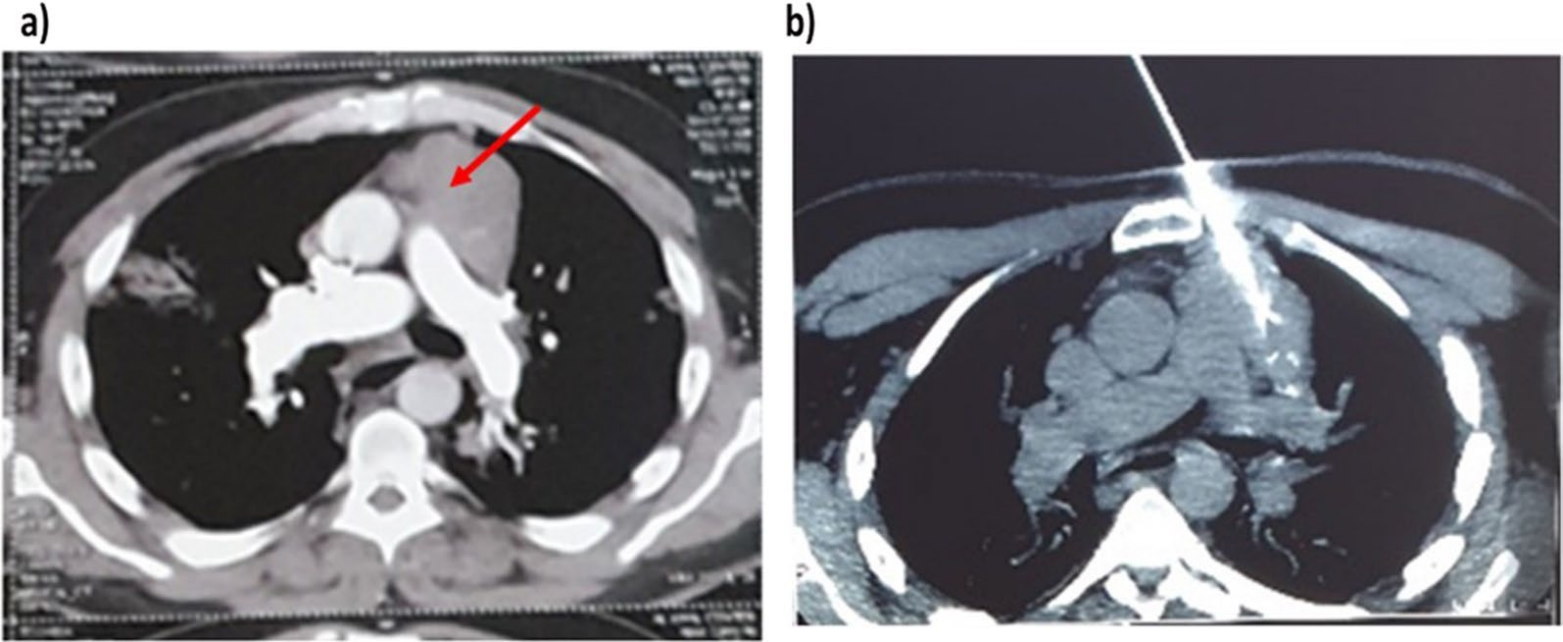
a Red arrow in the axial view of the chest’s computed tomography showing thymoma. b True cut needle biopsy
